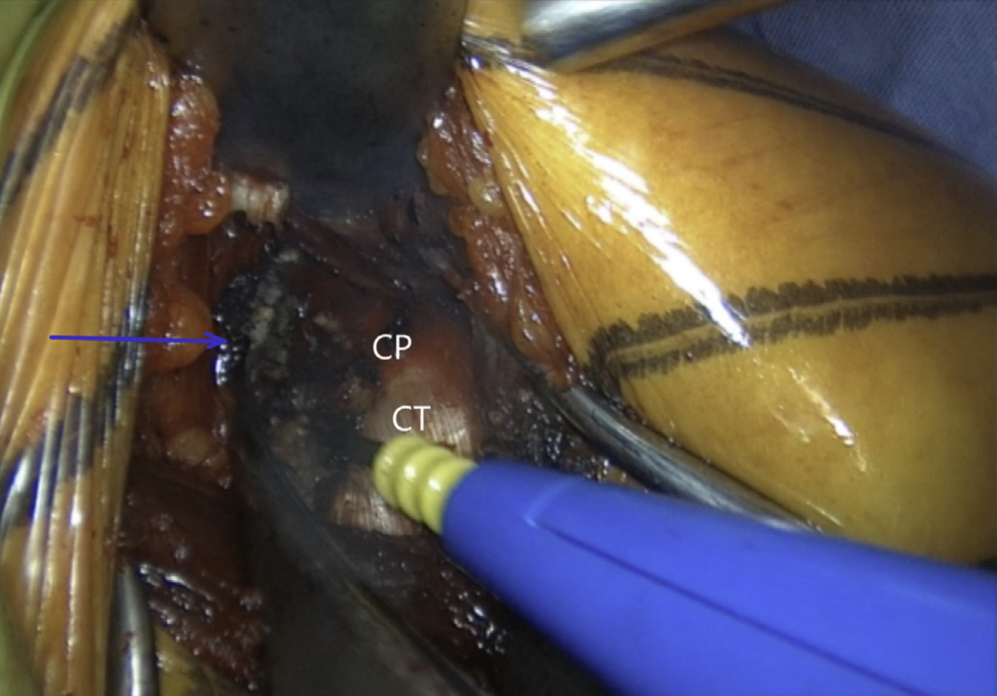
Left shoulder of a patient placed in a semi–beach-chair position. Pectoralis minor (blue arrow) is released from the coracoid process (CP) while the arm is in adduction and internal rotation. (CT, conjoined tendon.)
